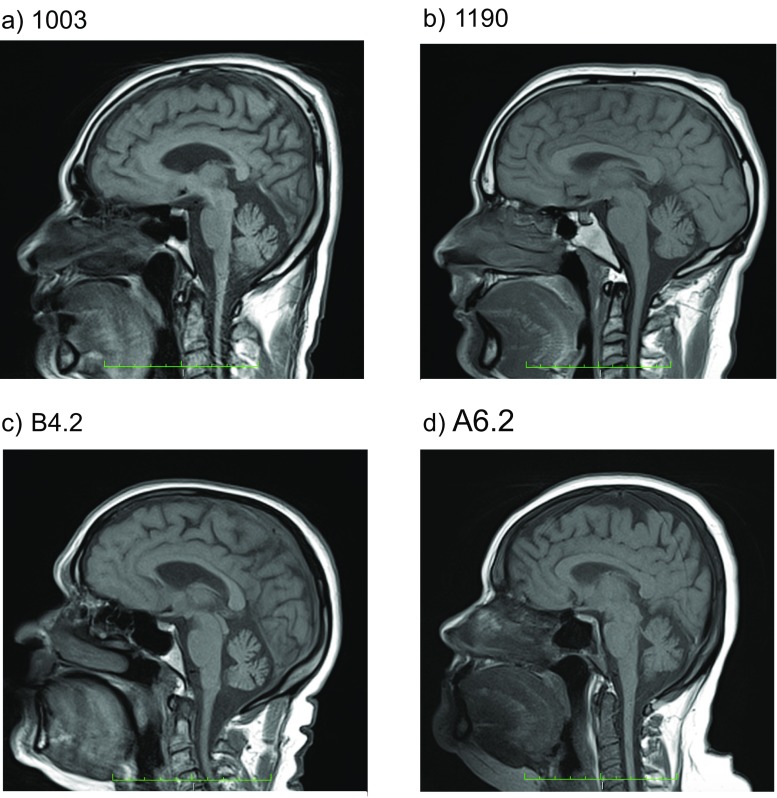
Midsagittal T1-weighted brain MRI of four CMT2B2 individuals showing mild cerebellar atrophy. a CMT1003. b CMT1190. c B4.2 (C). d A6.2
